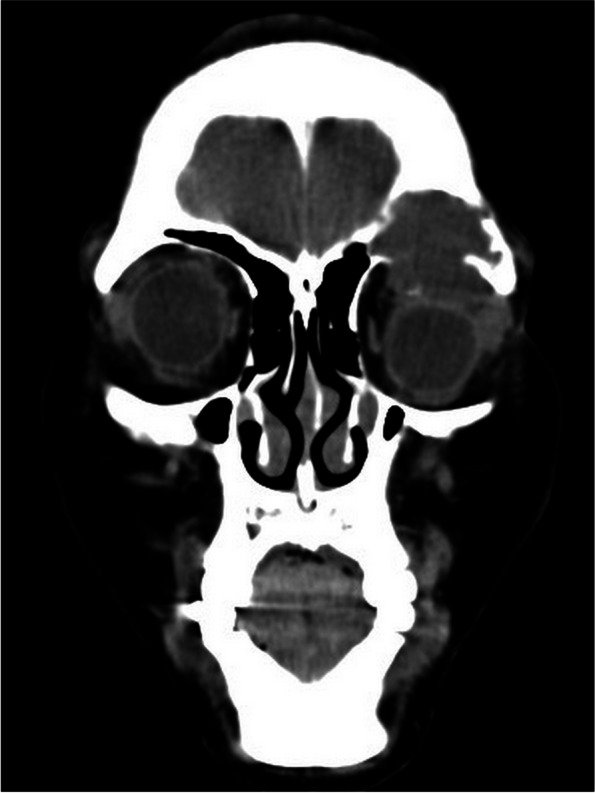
CT scan displayed a soft tissue mass in the left frontal sinus, eroding the floor of sinus, orbital roof, and extending into the superior orbital space
